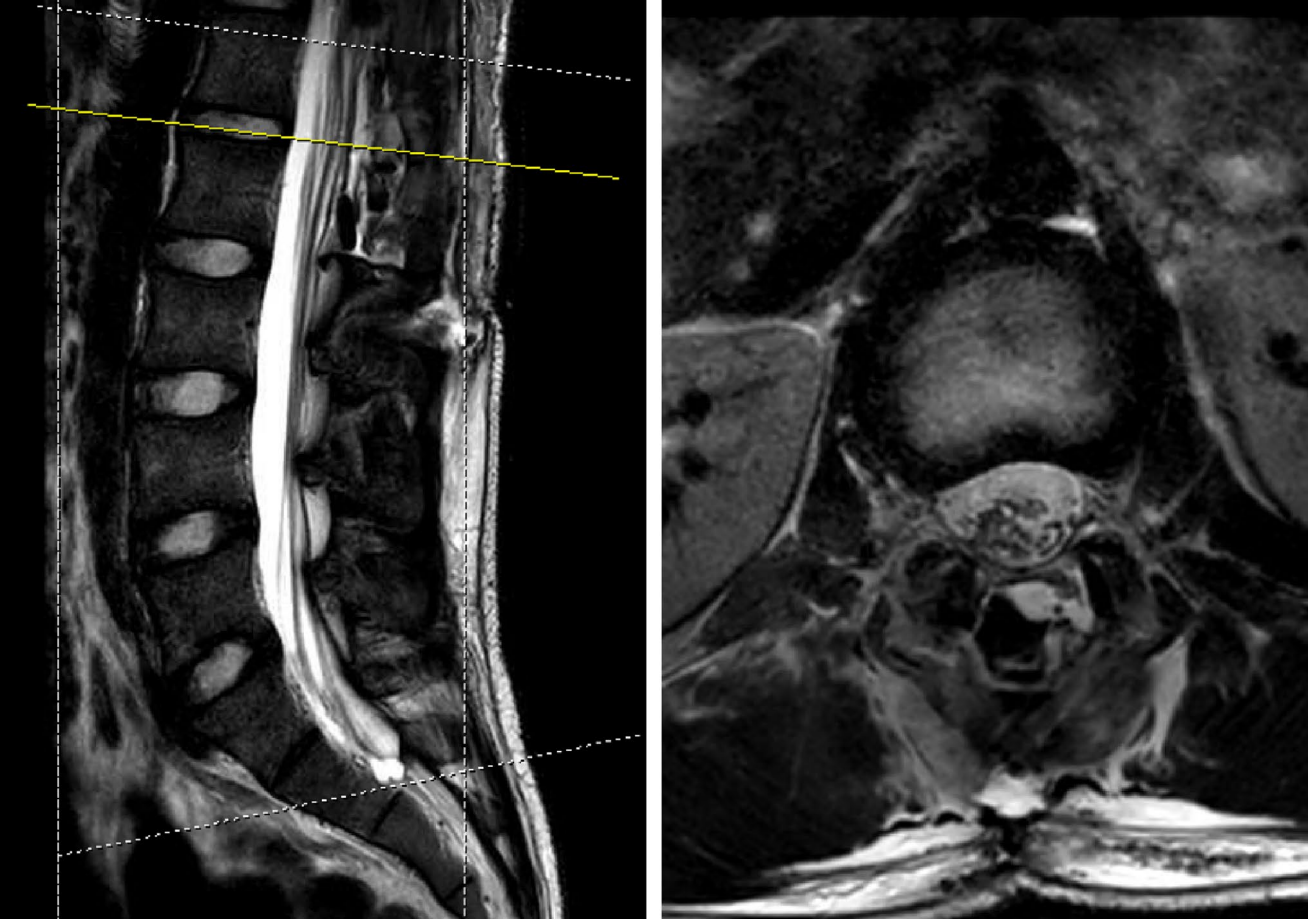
MRI lumbar spine without contrast obtained postoperative day 1. T2-weighted sagittal and axial images (left and right, respectively) demonstrating resection of lesion. The axial image is centered at the L1-L2 level
